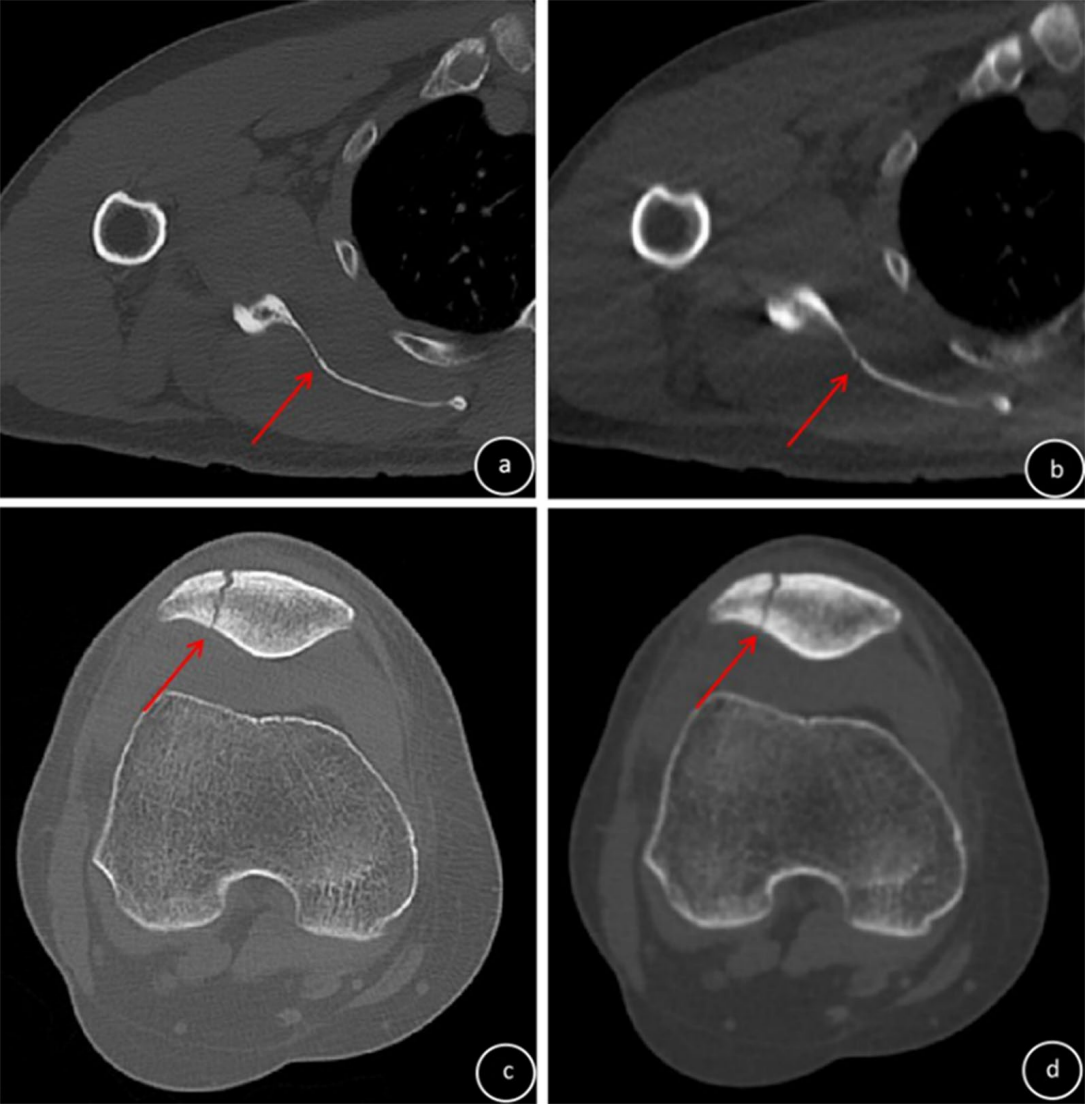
Diagnosis of non-displaced fracture of the shoulder (a, b) or knee (c, d) (red arrow). a and c: SD-CT images (subjective CT image quality and impact of subjective CT image quality on clinical decision-making, 5 points); b and d ULD-CT images (subjective CT image quality and impact of subjective CT image quality on clinical decision-making, 3 points)
